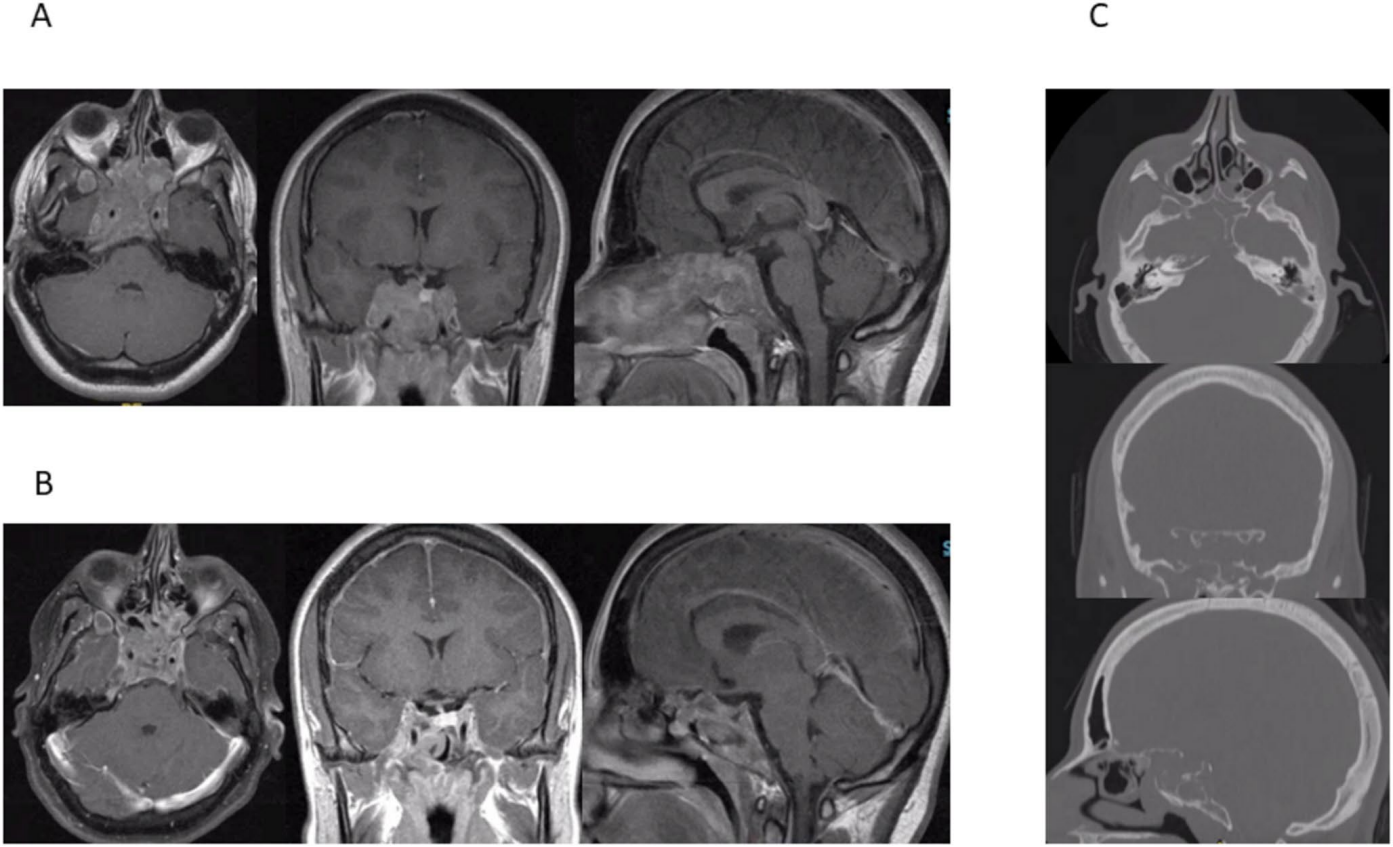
Imaging studies of patient 1 (19-year-old woman). A T1-weighted contrast enhanced MRI studies before re-administration of cabergoline therapy. The extensive pituitary tumor is widely invading the skull base, expanding to the right petrous bone, the cavernous sinus, the right sphenoid sinus and the clivus. B T1-weighted contrast enhanced MRI studies two months after re-administration of cabergoline therapy. The tumor has shrinked substantially. A small intratumoral cavity filled with air is visible. C CT imaging in bone window show the bony defects in the skull base (sella, clivus and sphenoid sinus wall)
